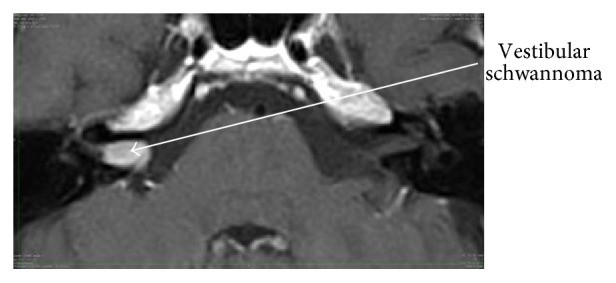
A vestibular schwannoma shown in a contrast-enhanced T1-weighted sequence.
